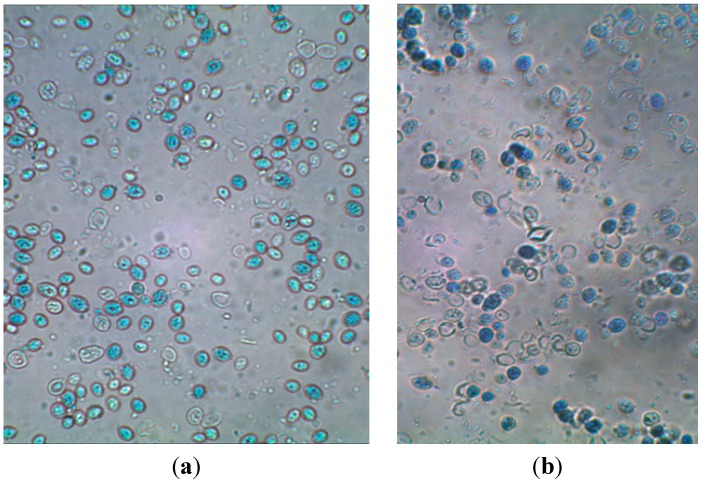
S. cerevisiae* cell wall preparations after cells disruption with the use of autolysis and ultrasounds (magn. 600×); (a) cells disrupted in deionized water; (b) cells disrupted in Tris-HCl buffer.
